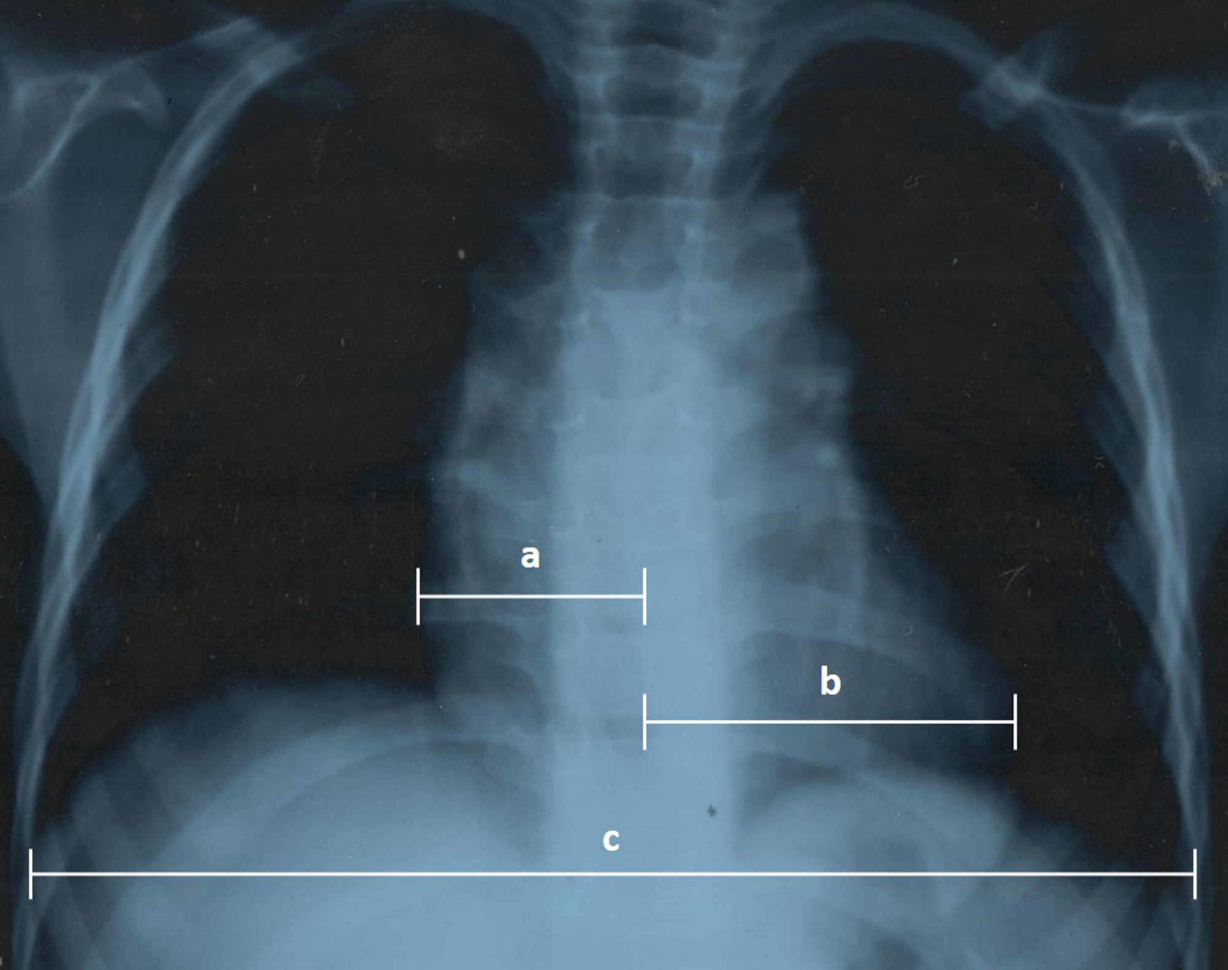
Chest X-ray posterior-anterior view showing cardiomegaly Cardiothoracic ratio = (a + b)/c
